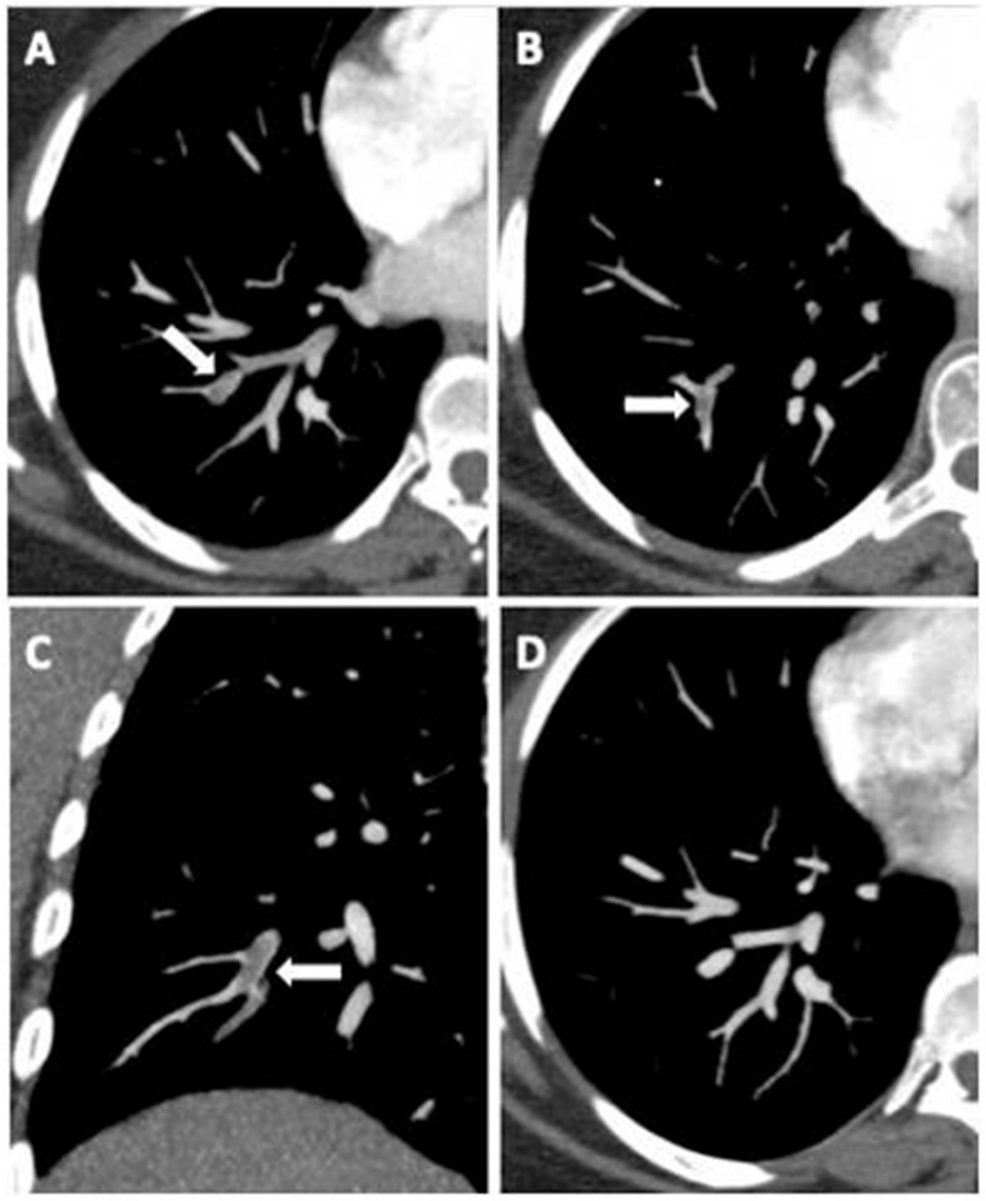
Axial CT images (A,B) and coronal multiplanar reconstruction (C) document partial centric and eccentric opacification defects in the right lower lobe basal segmental arteries (white arrows), suggestive of acute pulmonary embolism. Complete regression after anticoagulant therapy (D).
